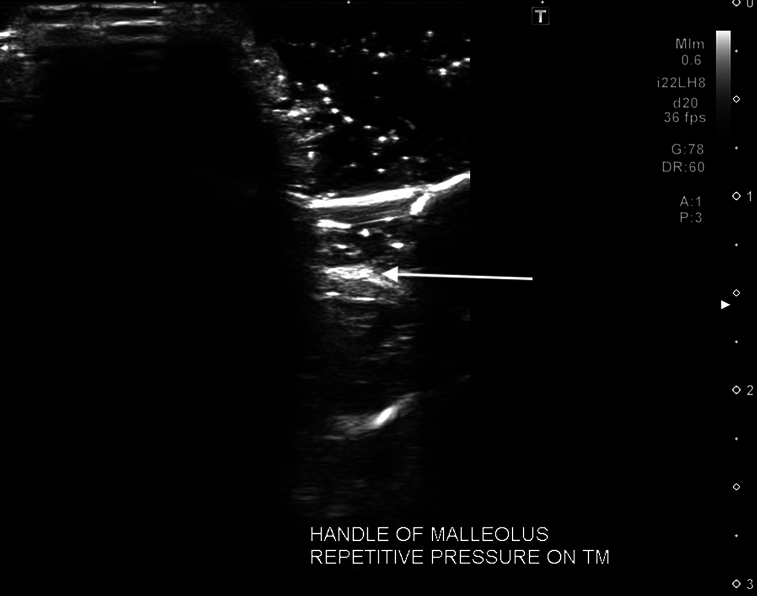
Sonogram (22 MHz) of the middle ear structures including the hammer of the malleus (arrow). The reflection and movement of the middle ear bones were demonstrated with manipulation and dynamic imaging.
